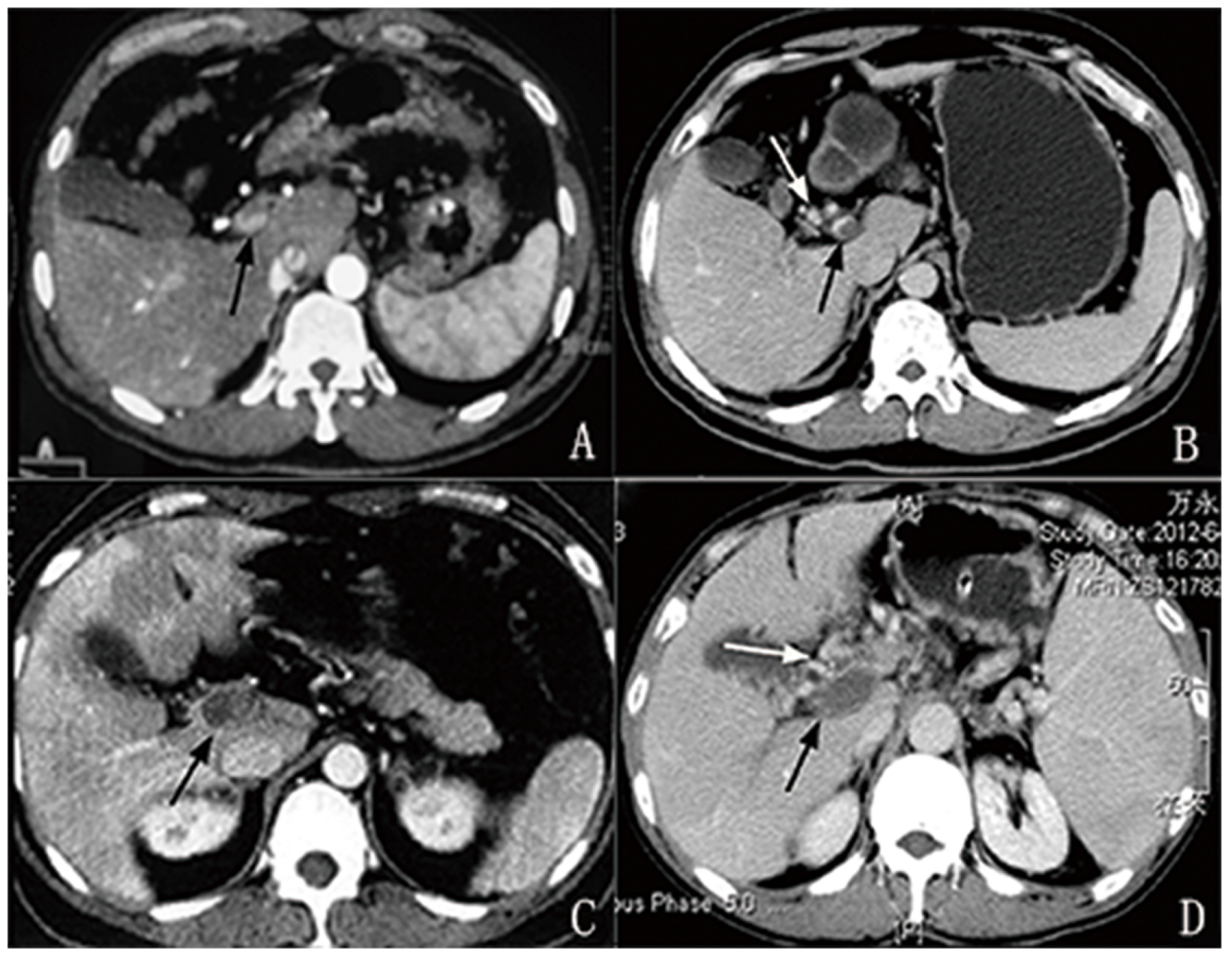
Cross-sectional CT image of portal vein thrombosis and cavernoma: A) partial PVT (black arrow) without cavernoma; B) partial PVT (black arrow) with cavernoma (white arrow); C) complete PVT (black arrow) without carvernoma and D) complete PVT (black arrow) with cavernoma (white arrow).
